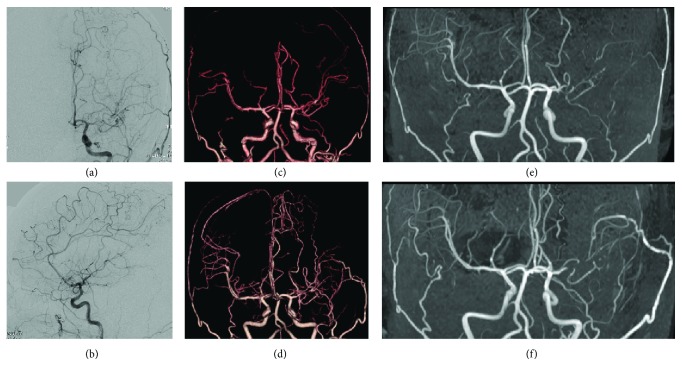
Pre- and postoperative cerebrovascular examinations. (a) and (b) Preoperative digital subtraction angiography showing narrowing in the M1 portion of MCA. (c) Preoperative 3D-CT angiography showing narrowing in the M1 portion of MCA. (d) Postoperative 3D-CT angiography showing the patency of STA-MCA anastomosis. (e) Preoperative magnetic resonance angiography (MRA) showing stenosis of M1 portion of left middle cerebral artery (MCA) and poor visualization of peripheral MCA. (f) Postoperative MRA showing the patency of STA-MCA anastomosis and improvement of blood flow in peripheral MCA.
